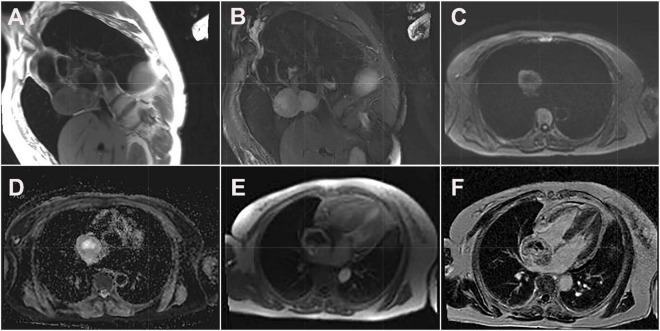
Cardiac magnetic resonance images of cardiac paraganglioma. (A) T1-weighted image; (B) T2 fat-suppressed image; (C) diffusion-weighted imaging; (D) apparent diffusion coefficient image; (E) first-perfused image; (F) delayed scans image.
